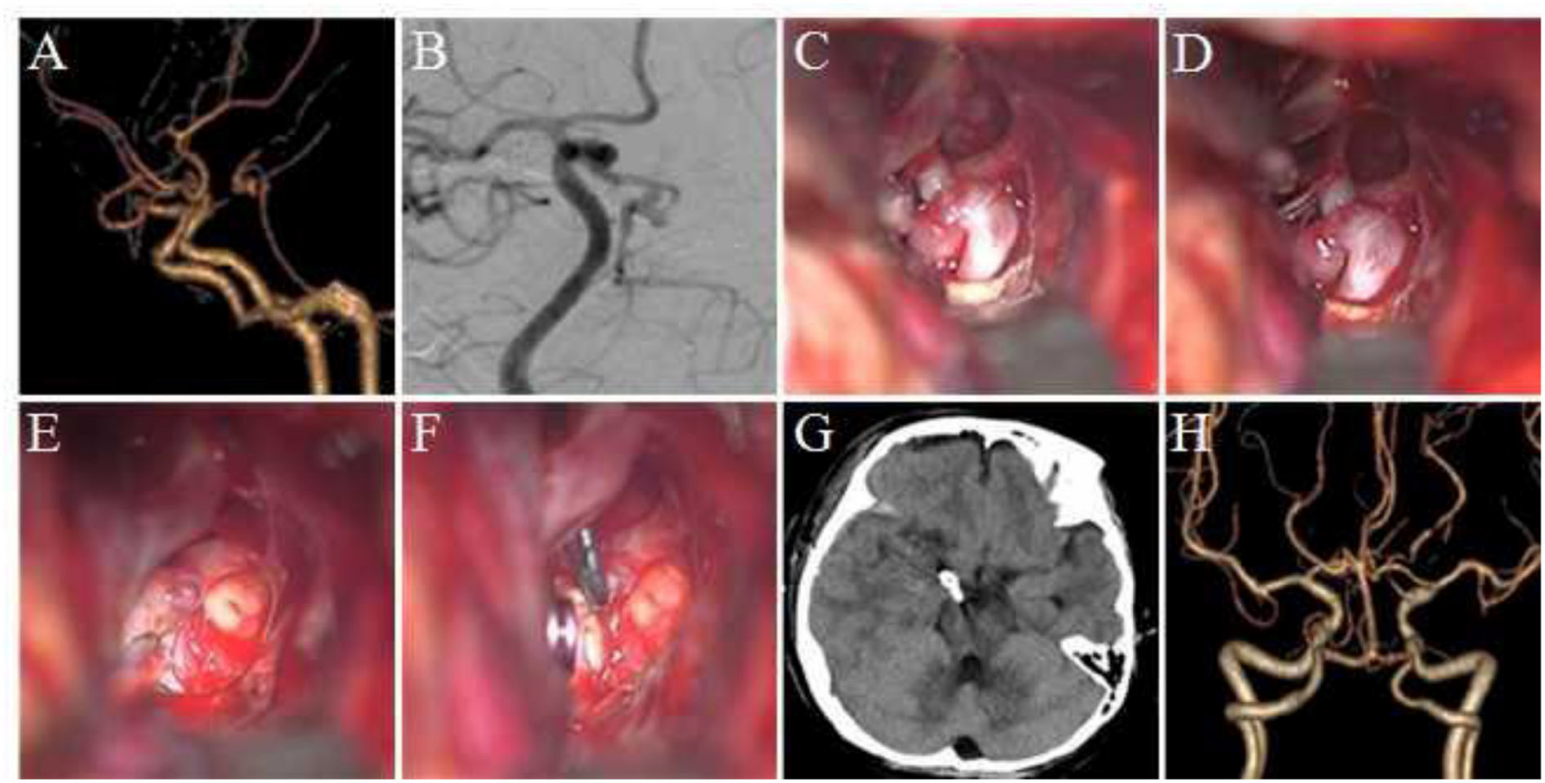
Clipping multiple aneurysms at the P1–P2 bifurcation of posterior cerebral aneurysm *via* the Dolenc approach. (A) Preoperative CTA revealed an aneurysm at the left posterior cerebral P1–P2 bifurcation with irregular shape and basilar artery and bilateral posterior cerebral artery stenosis. (B) DSA revealed two aneurysms at the bifurcation of P1–P2 of the posterior cerebral artery, exhibiting a lobulated shape. (C) During surgery, the P1–P2 bifurcation aneurysm could be seen in the lateral space of the internal carotid artery, which points to the posterior and medial side, and the oculomotor nerve and posterior communicating artery could be seen. (D) The optic nerve was pulled medially to increase the exposure range, and the P1 segment of the posterior cerebral artery, posterior communicating artery, and its perforating vessels could be seen. (E) Another aneurysm at the bifurcation of P1–P2 was exposed, pointing downward, and a part of the perforating vessels and oculomotor nerve from P2 could be seen. (F) Two aneurysm clips were used to clip the aneurysm. (G) A postoperative CT scan of the head. (H) The postoperative CTA revealed that the aneurysm was completely clipped with no residue and no vascular damage.
